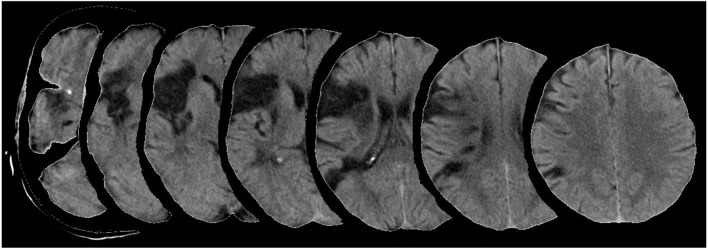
CT scans of patient GB after 3 months from stroke onset. Fronto-parietal hypointensity within the territory of the right middle cerebral artery is visible.
